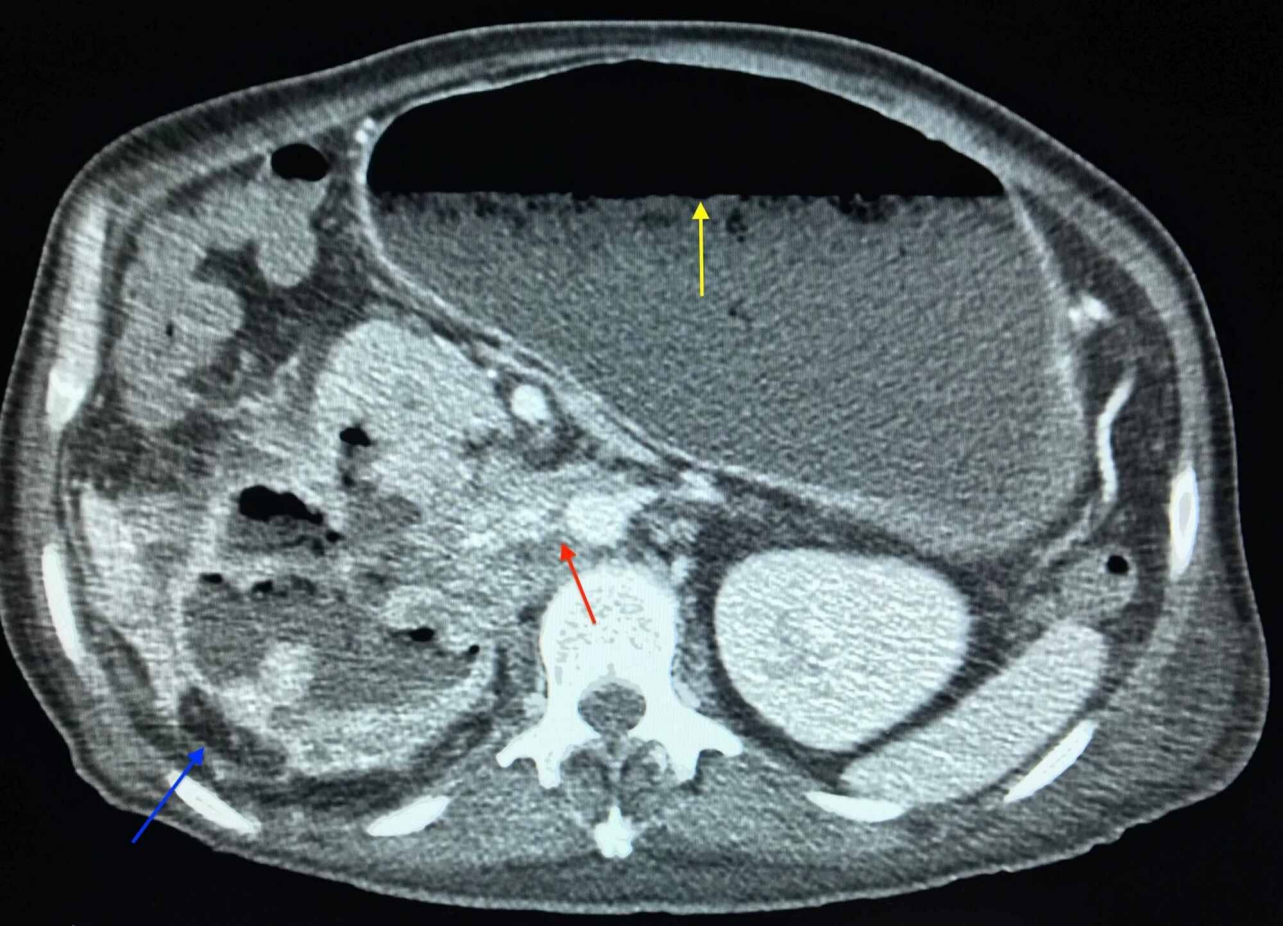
Filling defect of right renal vein/artery and posterior lateral nodular density of right kidney visualized on CT of abdomen, transverse plane Filling defect of right renal vein/artery is indicated by the red arrow. Posterior lateral renal nodular density is indicated by the blue arrow. The air-fluid level of the stomach is indicated by the yellow arrow.
